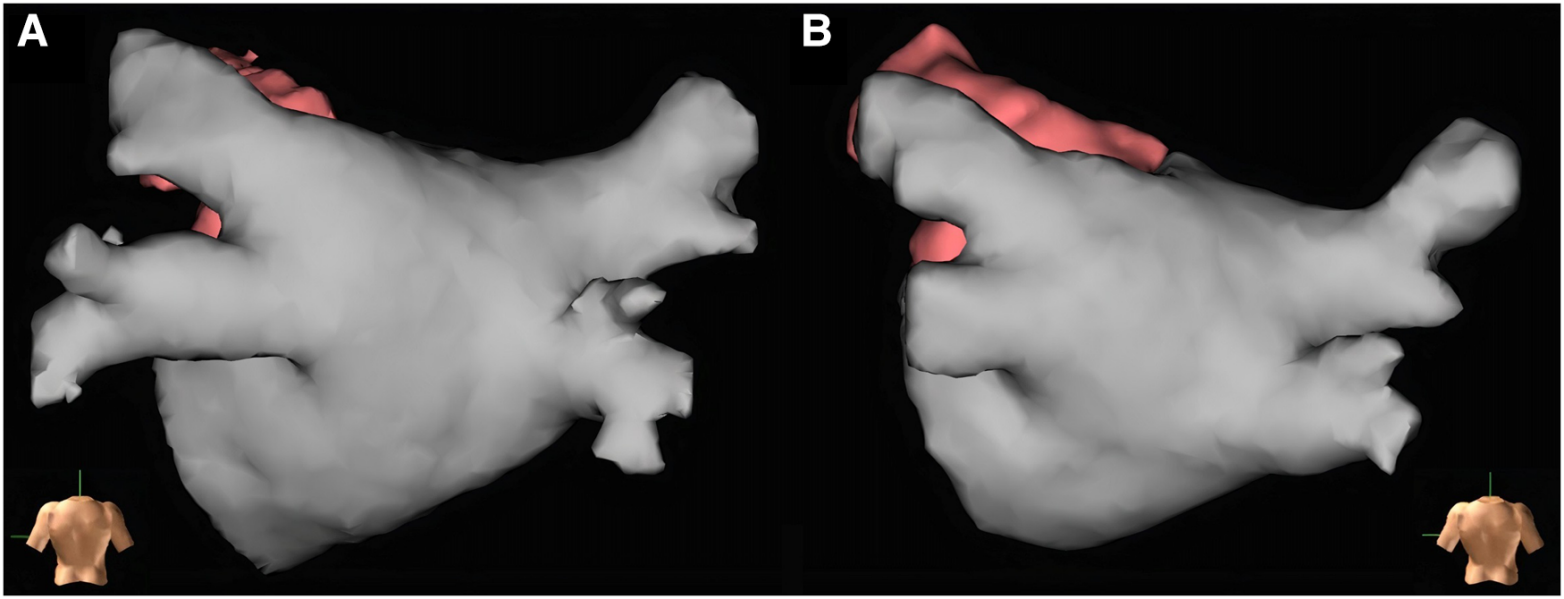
Left atrial reconstructions before radiofrequency catheter ablation for procedural planning based on left atrial contrast-enhanced CT and CMR BOOST bright-blood images created with EnSite Precision electroanatomical mapping system. Both reconstructions show the left atrium of the same patient (Heart and Vascular Center, Semmelweis University). Panel (A): Reconstruction based on left atrial contrast-enhanced CT images. Panel (B): Reconstruction based on left atrial CMR BOOST bright-blood images.
